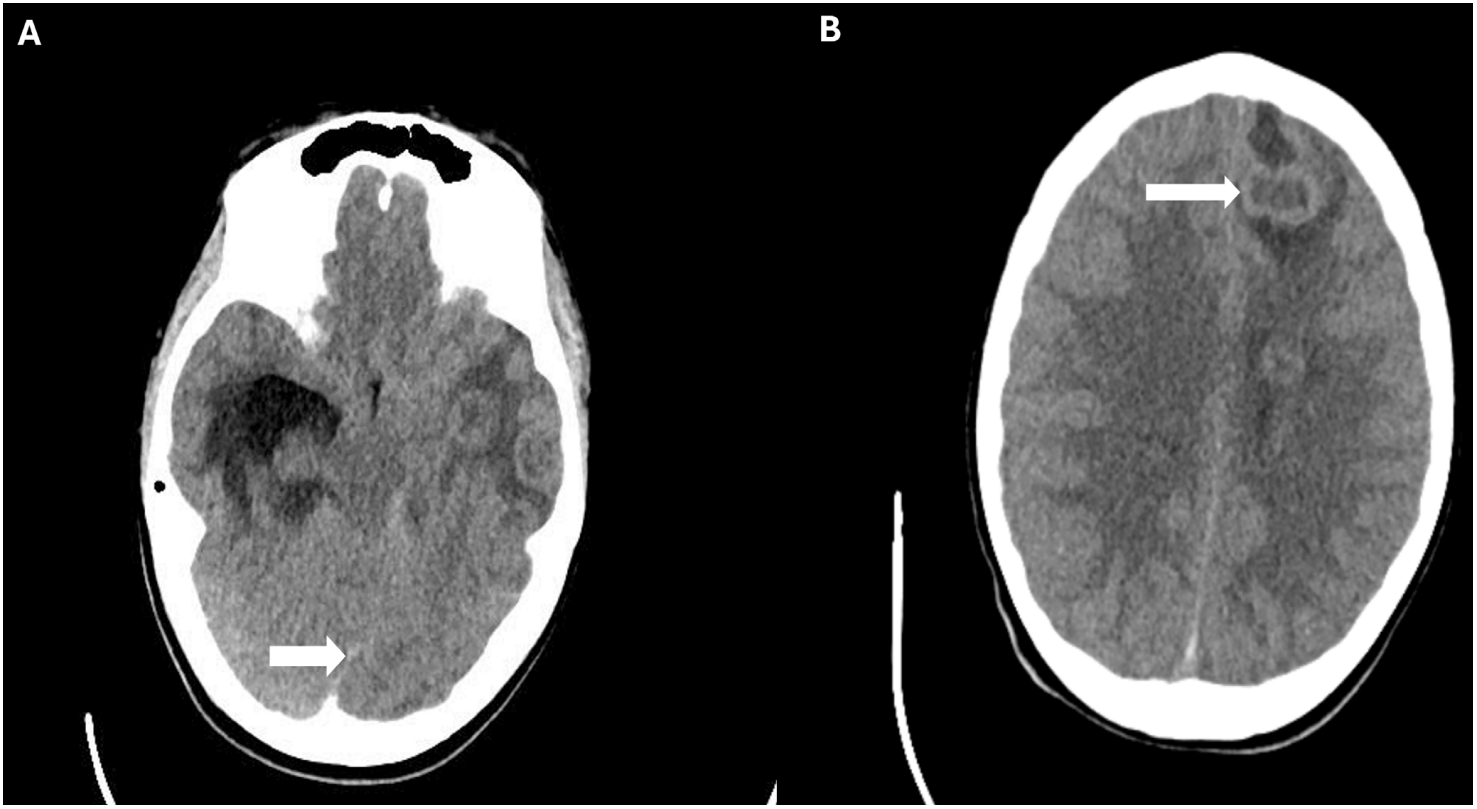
A: Axial head CT image showing the midline shift (white arrow); B: Axial head CT image showing multiple brain abscesses.
